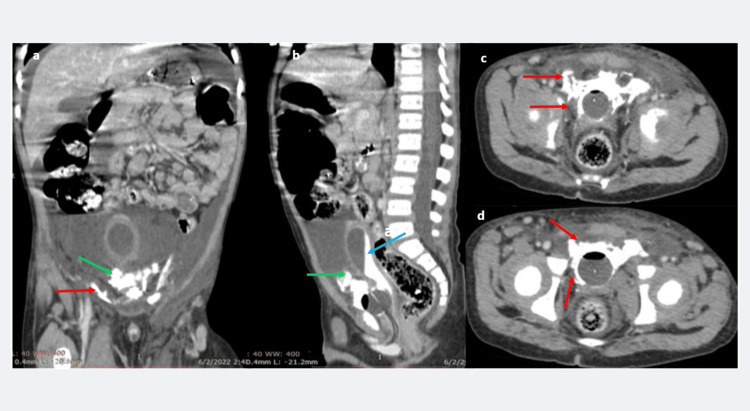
a-d: CECT scan (delayed phase, 30 minutes) images reveal, extravasation of contrast around the anterior bladder wall into the pelvic peritoneum (green arrow in a, b), a contracted and poorly distending urinary bladder is seen (blue arrow in b). Additionally, extensive extravasation of contrast is seen into the extraperitoneal, extra-vesical space (red arrows in a, c, d). This phase of the study demonstrates the combined intraperitoneal-extraperitoneal urinary bladder injury. CECT: contrast-enhanced CT scan
